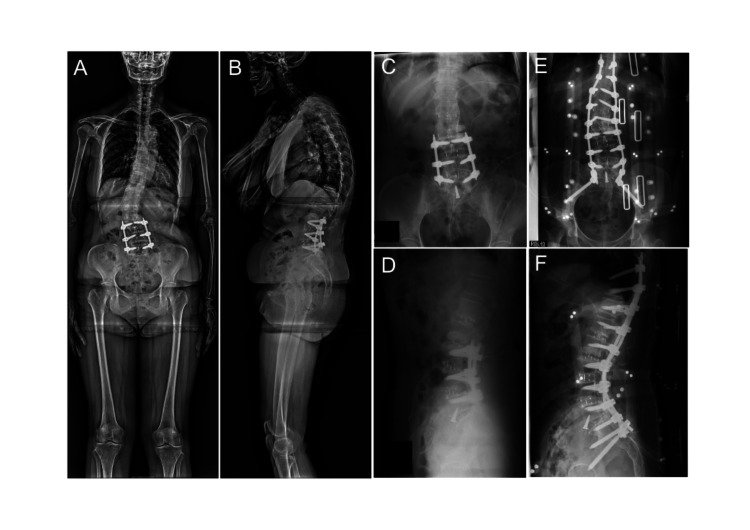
Radiographs before and after oblique lumbar intervertebral fusion and posterior fixation via percutaneous pedicle screws. Adult spinal deformity following the first oblique lumbar intervertebral fusion (OLIF) of L3/4 and L4/5 was observed. The angle between the L5 and S1 was originally 12°, changing to 18° after OLIF, including the L5/S1 level, and 23° after posterior fixation via percutaneous pedicle screws. Images taken (A, B) before OLIF, including the L5/S1 level, (C, D) after OLIF, (E, F) after posterior fixation via percutaneous pedicle screws. (A, C, E) anterior-posterior projection, and (B, D, F) lateral projection.
